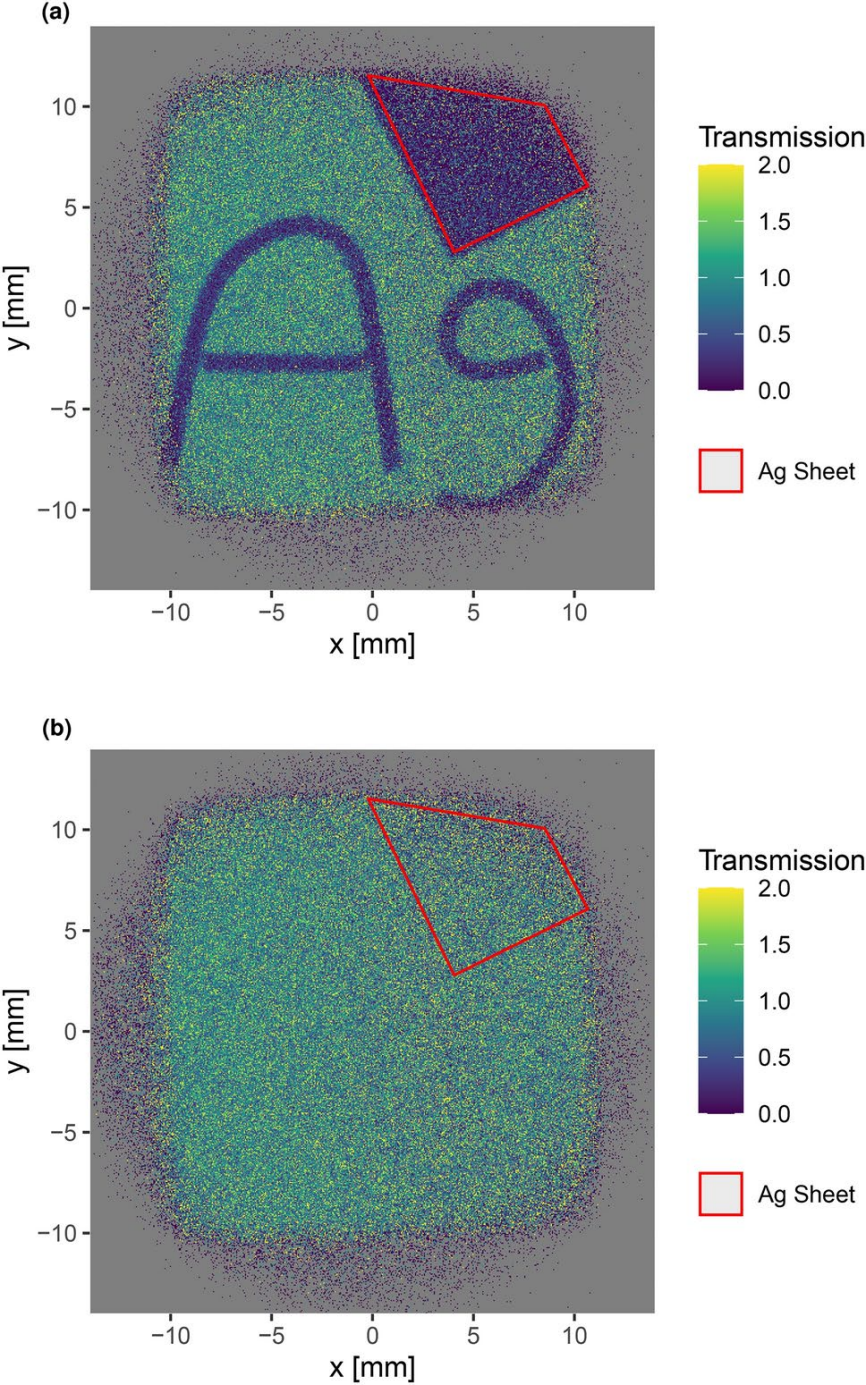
Radiograph of the test sample in the lowest energy resonance interval (a) and in an off-resonance region (b). The samples are clearly visible in (a), including small details, but almost completely invisible in (b). The silver sheet region used to produce the data displayed in Fig. 7 is shown in red. The grey region along the edges of the images is outside the neutron beam.
